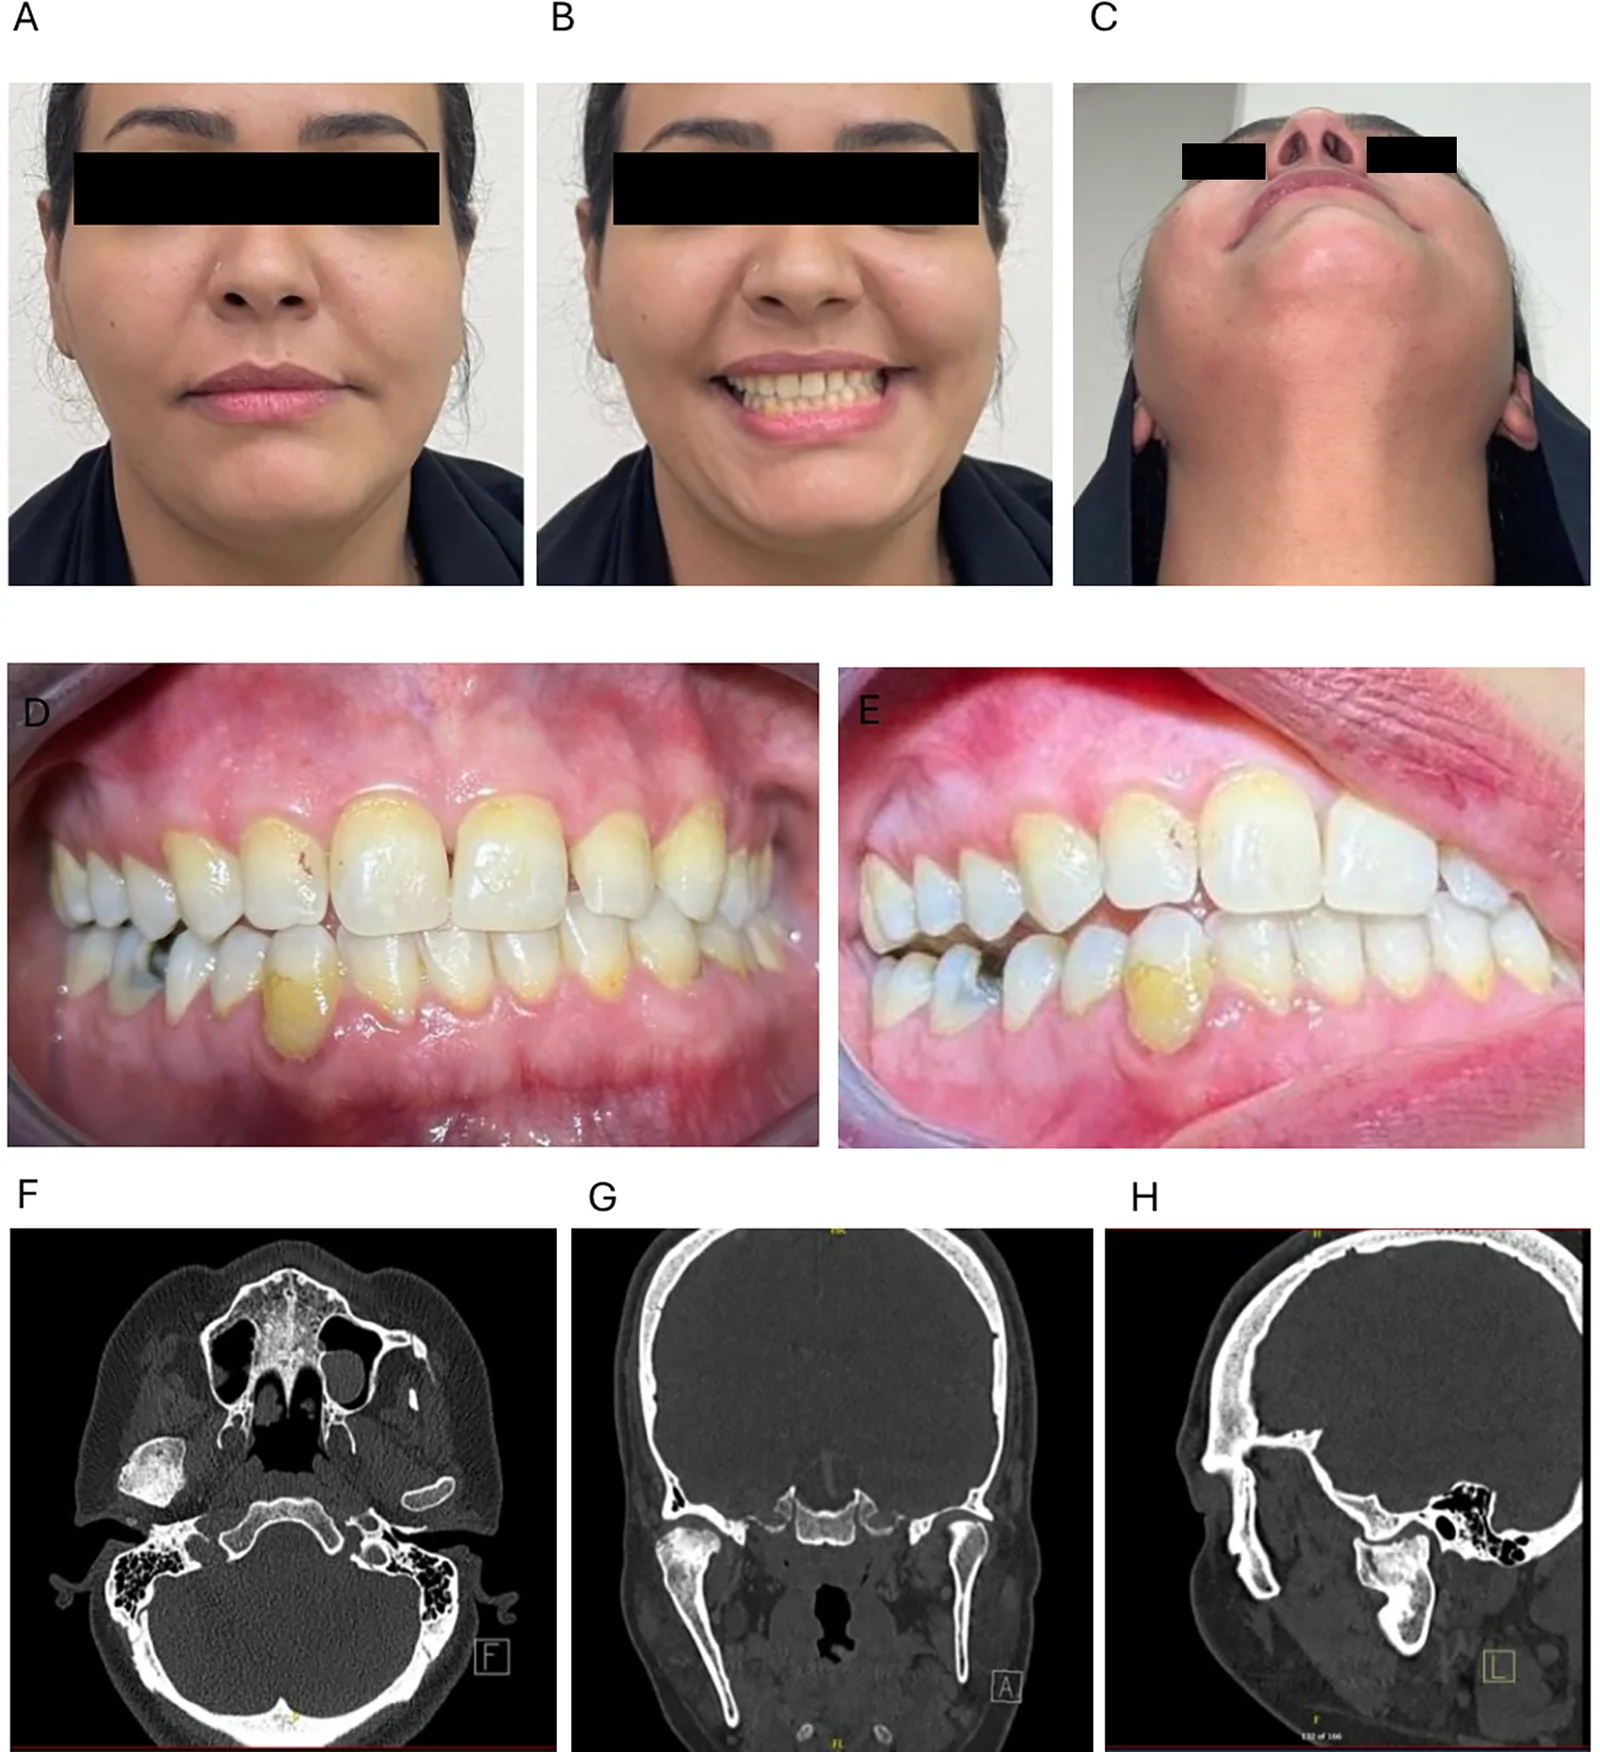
Photographs of the patient depicting FA with chin deviation to the left: (A) frontal view at rest, (B) frontal view with a smile, (C) submental (worm's eye) view, (D) canting of maxillary and mandibular occlusal planes downward on the right side with a mandibular midline shift to the left, (E) open bite in the right posterior segment, (F) CT axial plane, and medial extension of the CO evident on the (G) coronal plane and (H) sagittal plane.
